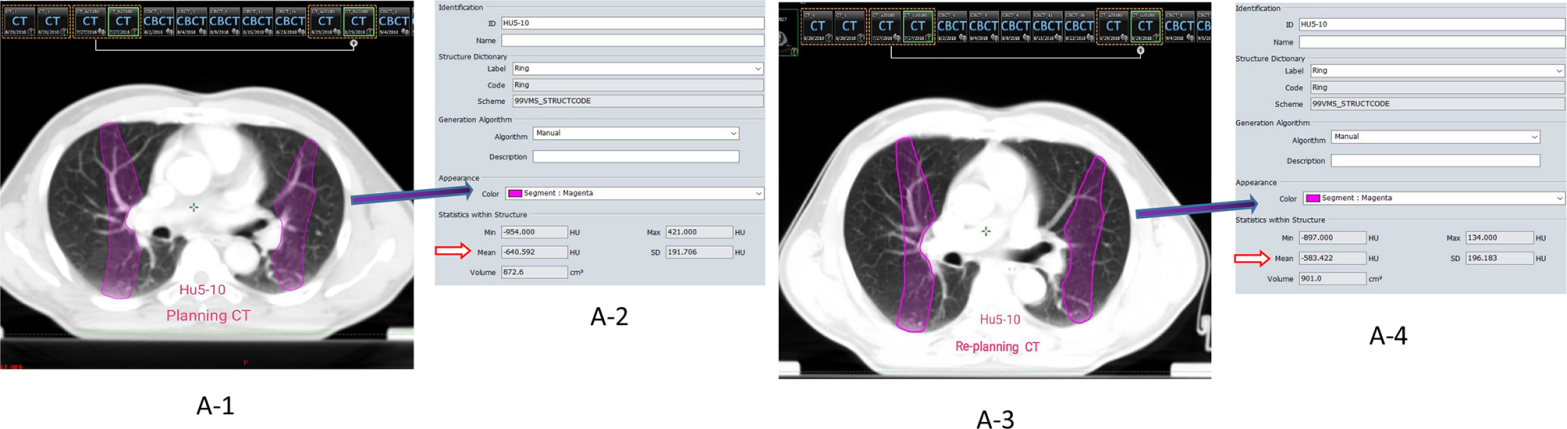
(A_1-4_) The dose gradient interval of HU_5-10_ was selected as an example. ①A-1/A-3 represent the images of the planning CT and the re-planning respectively (Rigid Registration); ②A-2/A-4 represent the parameters of the gradient interval of HU_5-10_, respectively (MeanHU: mean density value); ③Calculation of density difference (ΔHU) = Planning CT (HU_mean_) − Replanning (HU_mean_).
